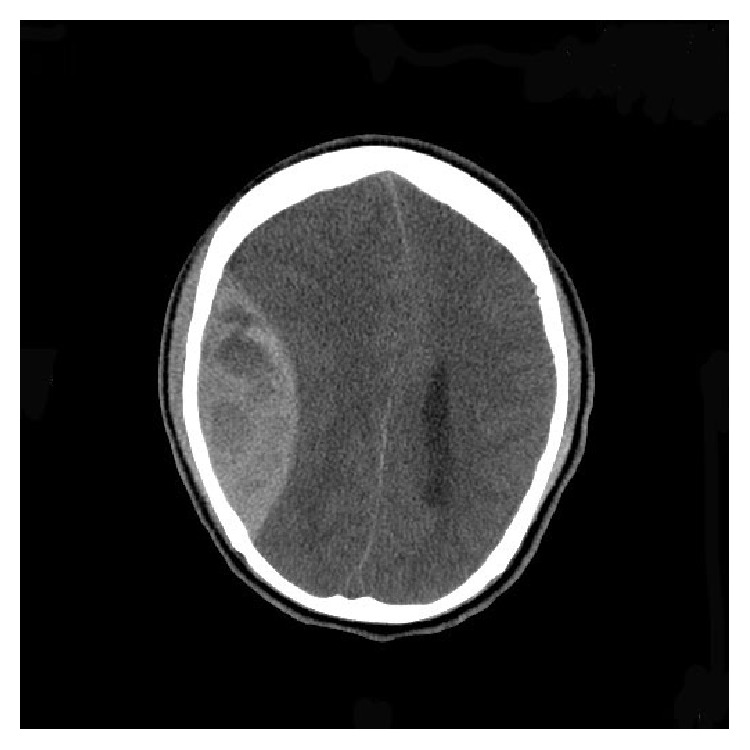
Preop CT showing right large epidural hematoma with midline shift.
